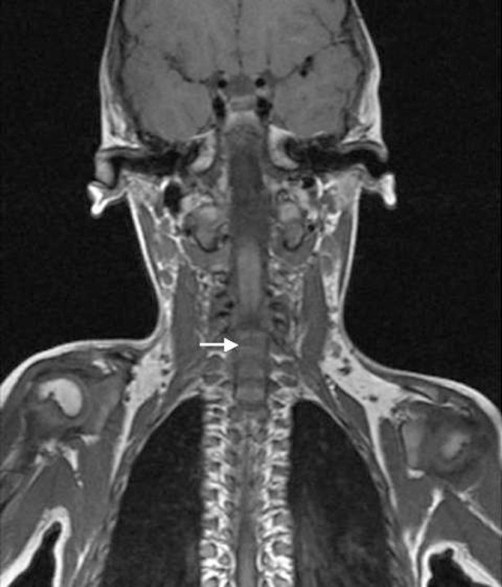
Coronal T2-MRI showed significant defective ossification of the vertebral bodies (arrow).
